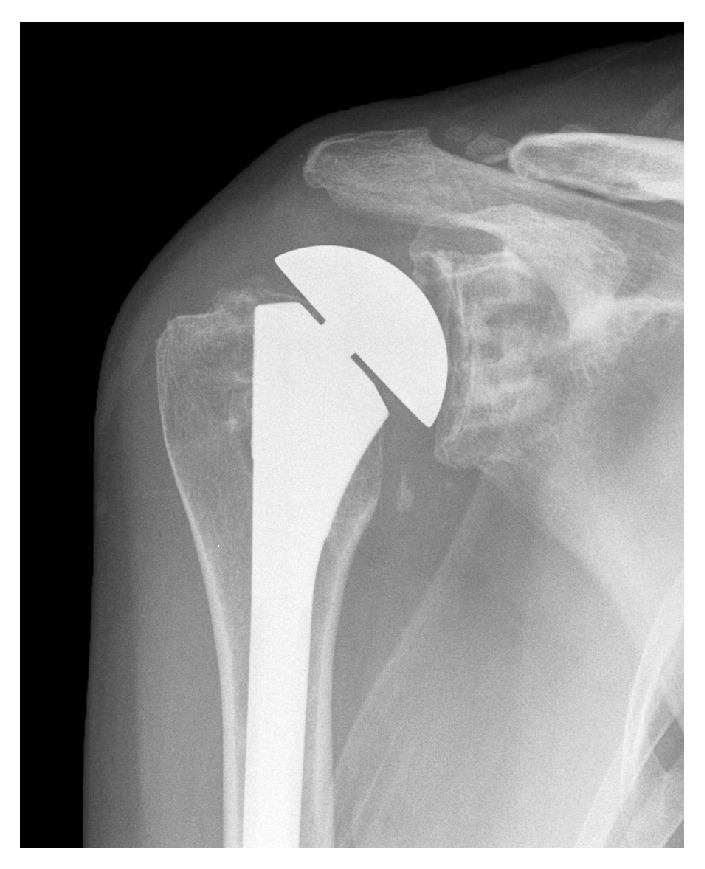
Anterior-posterior radiograph of the patient's right shoulder taken seven months after the index TSA. The distance between the acromion and humeral head is not narrowed in this image. This contrasts with the later radiograph shown in Figure 3 where this distance is significantly narrowed (which suggests weakness of the superior rotator cuff).
